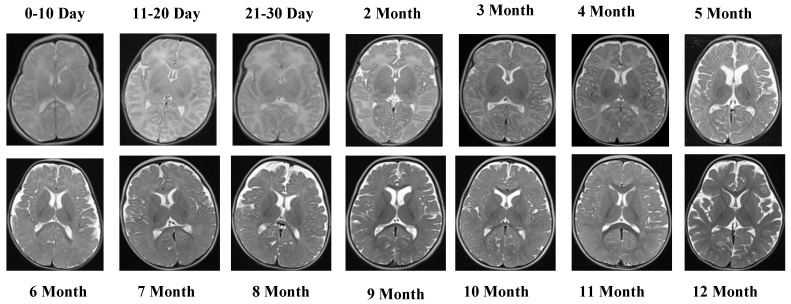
MRI slices from the T2 Female sequence.
